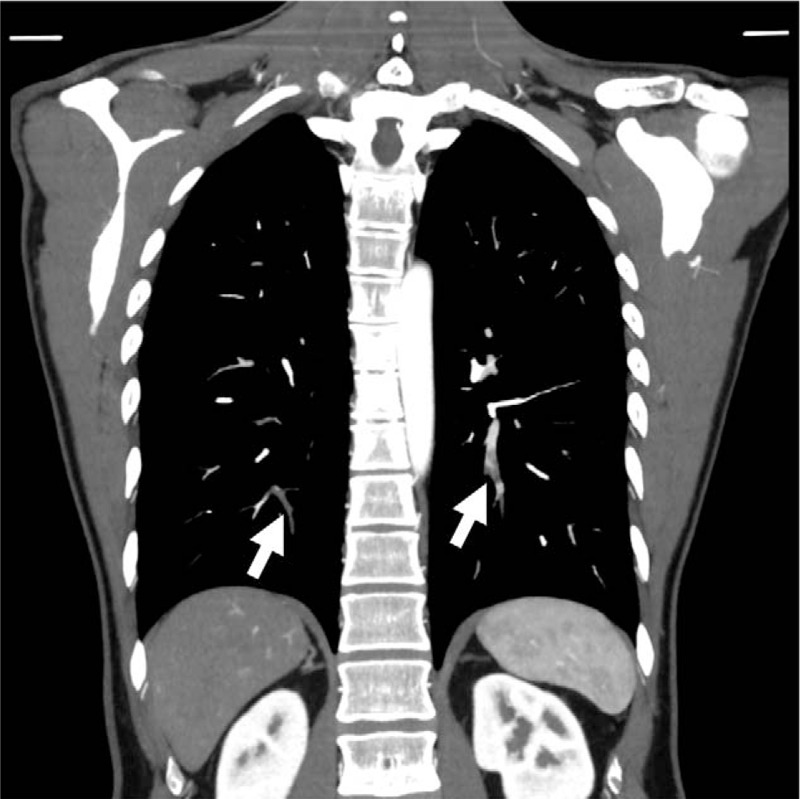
Contrast enhanced computed tomography on admission showing thrombus in the bilateral pulmonary arteries (arrows).
